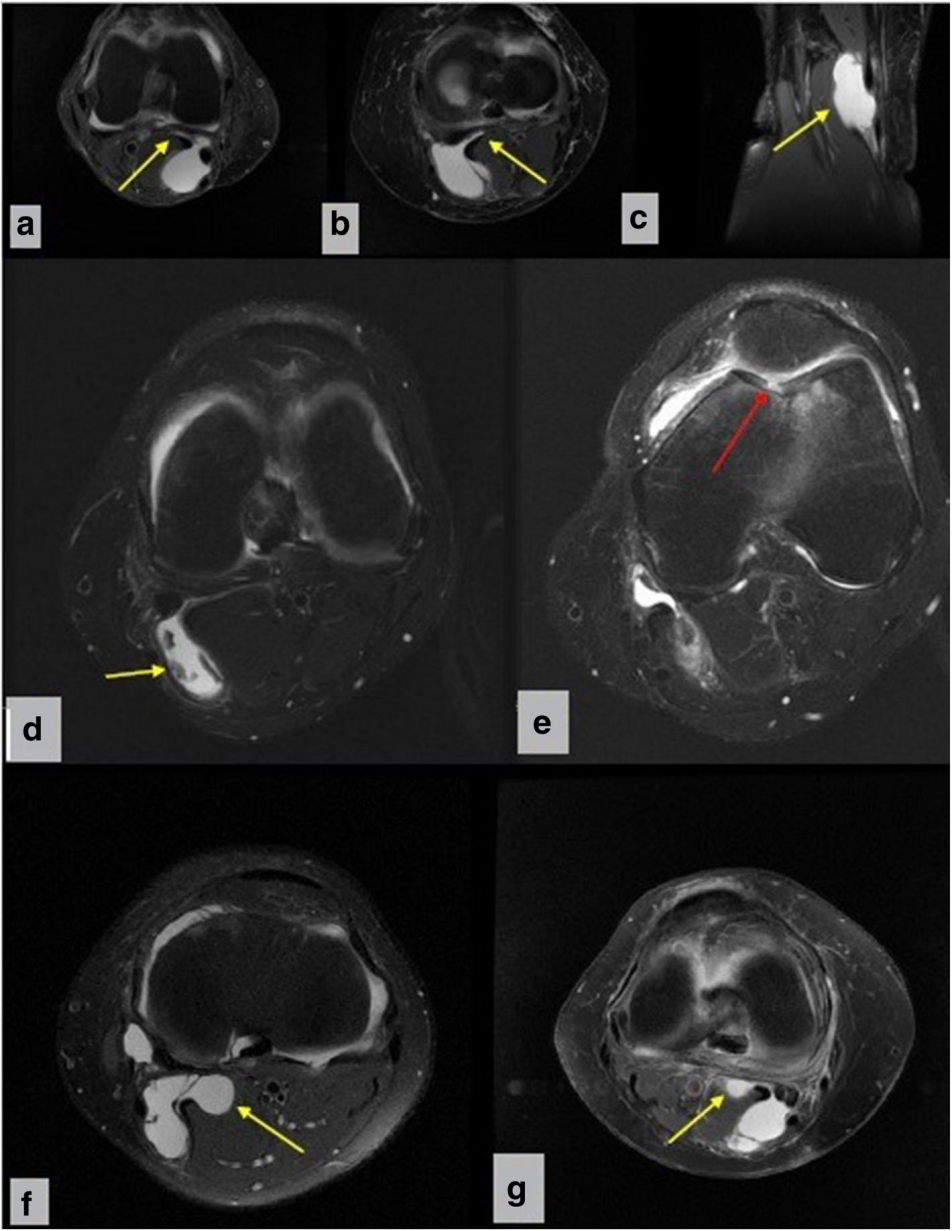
Selected sample MRI studies which demonstrate the typical peduncle and cartilage lesions. a, b- Different sample cases, axial images; notice the peduncle (*yellow arrow*) of the cyst adherent to posterior capsule. c- Coronal T2 weighted image that shows cyst (*yellow arrow*) located near the gastrocnemius medial head. d. Axial image that shows intracystic loose bodies (*yellow arrow*), originated from trochlear fissure that moved through the valve and trapped, a typical case that demonstrate the loose bodies in the cyst and effusion. e. The same case with figure d, The arrow (*red*) shows trochlear cartilage fissure that generate chondral debris. f g. Different sample cases, axial images; notice the cyst (*yellow arrow*) adherent to posterior capsule
